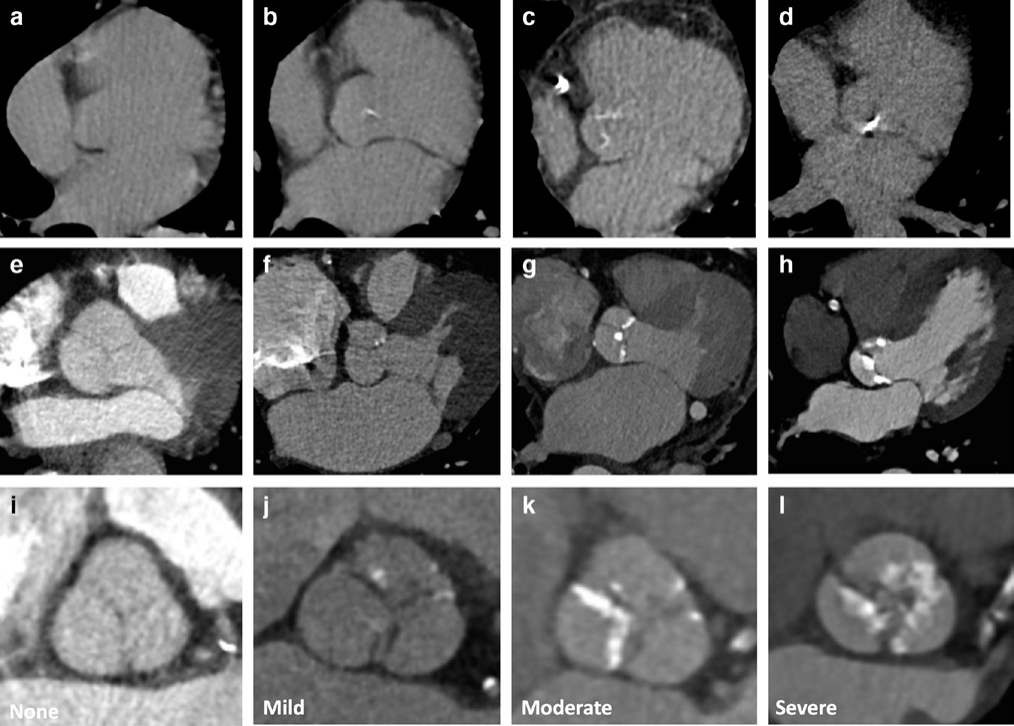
Aortic valve calcification severity. Images show different severities of aortic valve calcification from different patients on non-contrast (A–D) and contrast enhanced CT (E–L). Multiplanar reformats can help to differentiate aortic valve calcification from annular or aortic calcification (I–L). Images show no calcification (A, E, I) and mild (B, F, J), moderate (C, G, K) and severe (D, H, L) aortic valve calcification.
